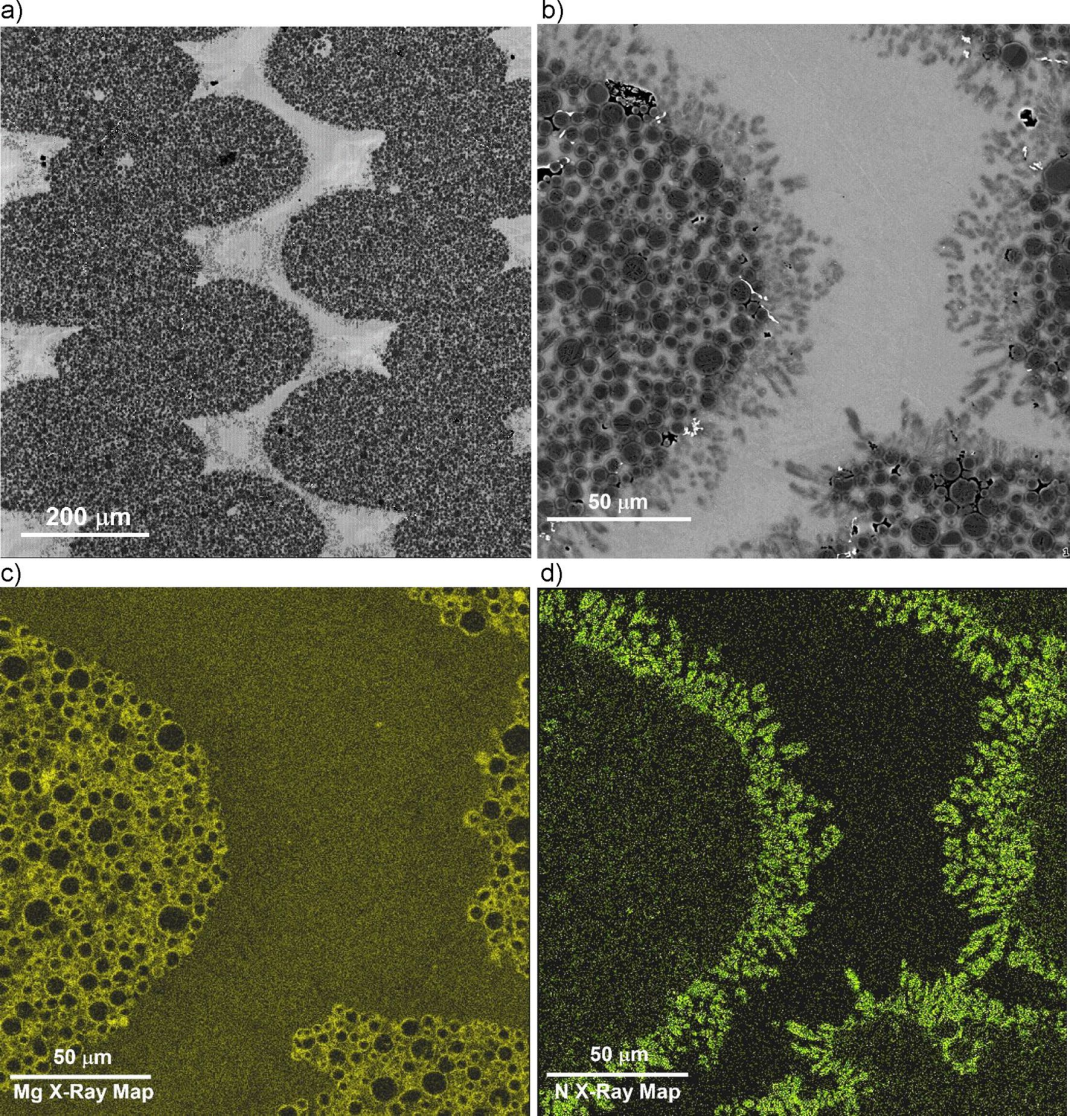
Typical microstructure of a particulate reinforced (80% infill) AMC manufactured using 3D printing technology. (a) BSE image; (b) high magnification view used for EDS; (c) Mg characteristic X-ray map; (d) N characteristic X-ray map.
